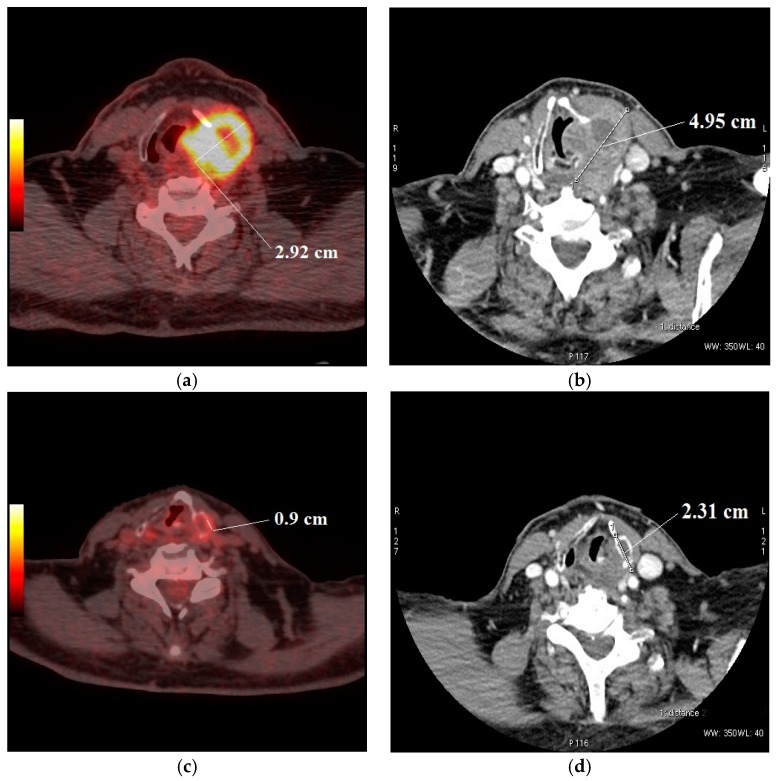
Contrast-enhanced CT and ^18^F-FDG PET/CT were performed at staging and after induction chemotherapy in patients with hypopharyngeal carcinoma. Tumor short axis alteration: (a,c) ^18^F-FDG metabolic uptake long axis before treatment 2.92 cm and after ICT—0.9 cm; (b,d) CT shows tumor short axis before treatment—4.95 cm and after treatment—2.31 cm.
